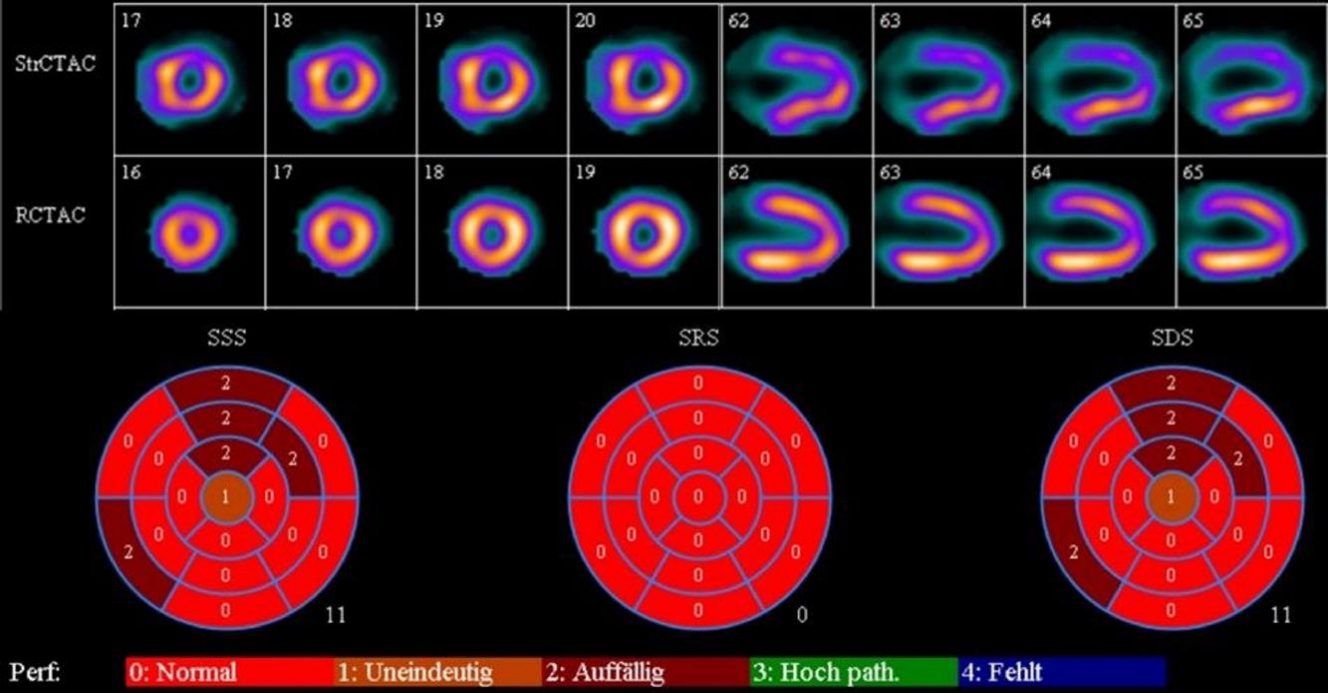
Myocardial perfusion single-photon emission computed tomography (SPECT). Under stress (StrCTAC), there is reduced uptake in the anterior wall (domain of the left coronary artery) compared to rest (RCTAC) as seen in axial (17–20/16–19) and sagittal (62–65) views. SDS is 11, which indicates that ∼16% of the myocardium is affected by reversible stress-induced ischemic changes. StrCTAC, stress conventional tomographic angular correction; RCTAC, rest conventional tomographic angular correction; SSS, summed stress score; SRS, summed rest score; SDS, summed difference score.
